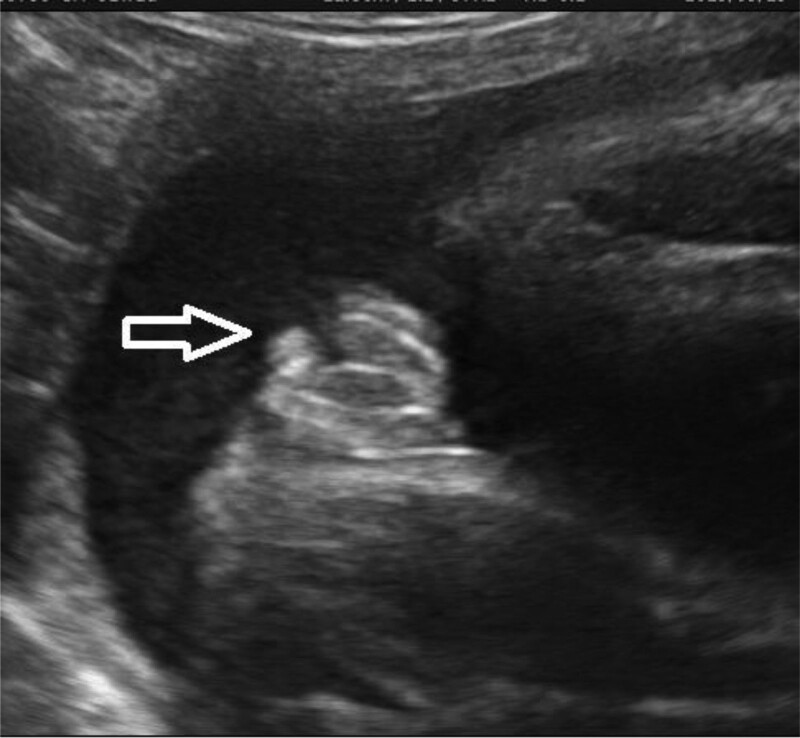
Ultrasound examination of the fetus. At 32^+1^ wk of pregnancy, ultrasound showed the female genitalia, the labia were visible, and an iso-echoic mass of about 8 mm × 5 mm was protruding from the genitalia.
